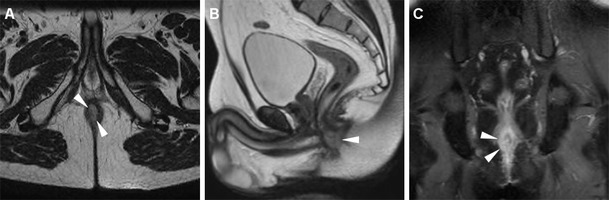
A 40-year-old MSM with bioptic diagnosis of SCAC. Axial (a) and sagittal (b) T2-weighted images show 2-cm hyperintense nodule contained within the internal sphincter muscle, intensely enhancing as seen on post-contrast fat-suppressed coronal T1-weighted image (c), consistent with T1 tumour (*arrowheads*)
